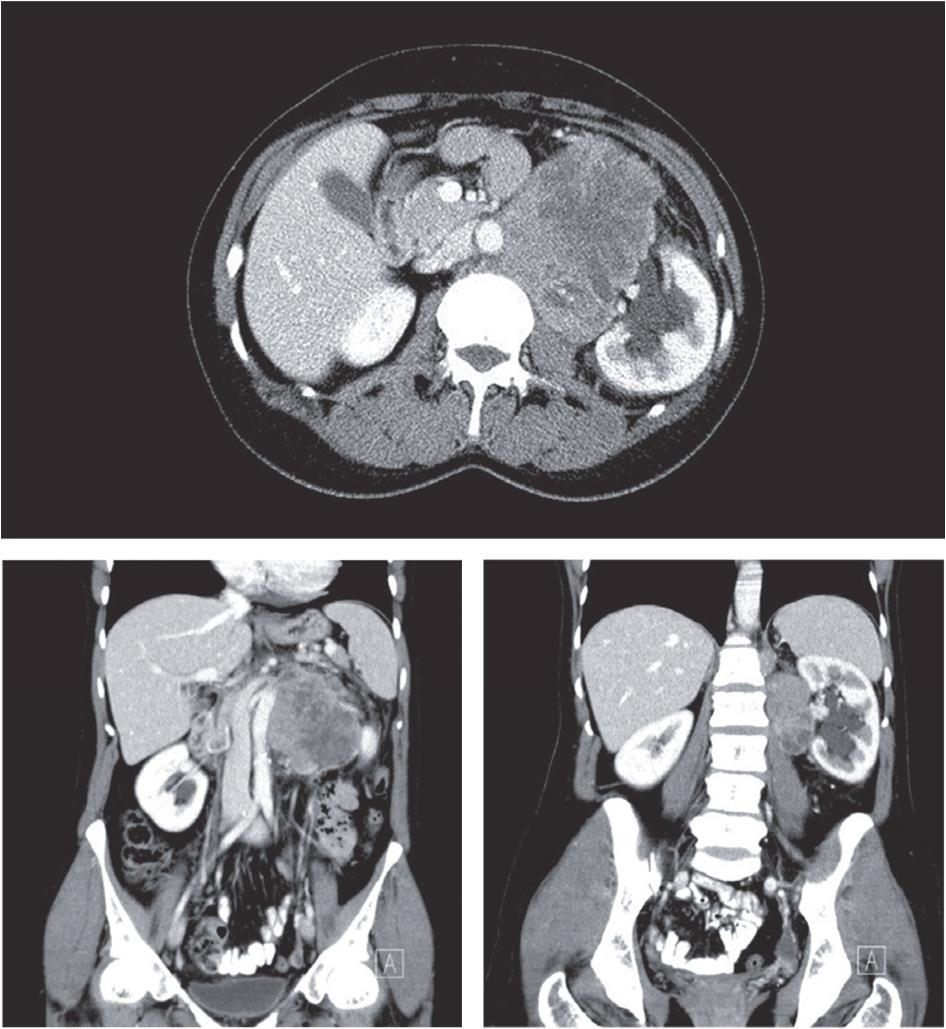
Enhanced computed tomography showing retroperitoneal tumor, interposed between the aorta and the left kidney and axial and coronal plane.
